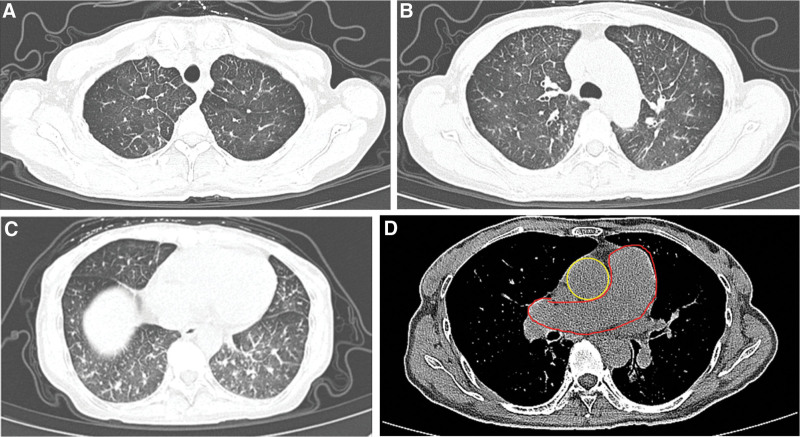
Ground-glass opacity, interlobular septal thickening and enlarged pulmonary artery in computed tomography.
